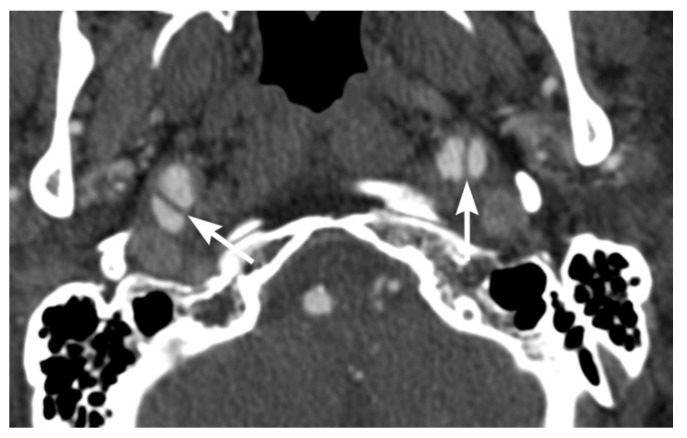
68-year-old male with bilateral BCVI and major thoracic injuries following a motor vehicle accident. Axial multidetector CT angiographic image shows bilateral distal cervical internal carotid artery dissection.
